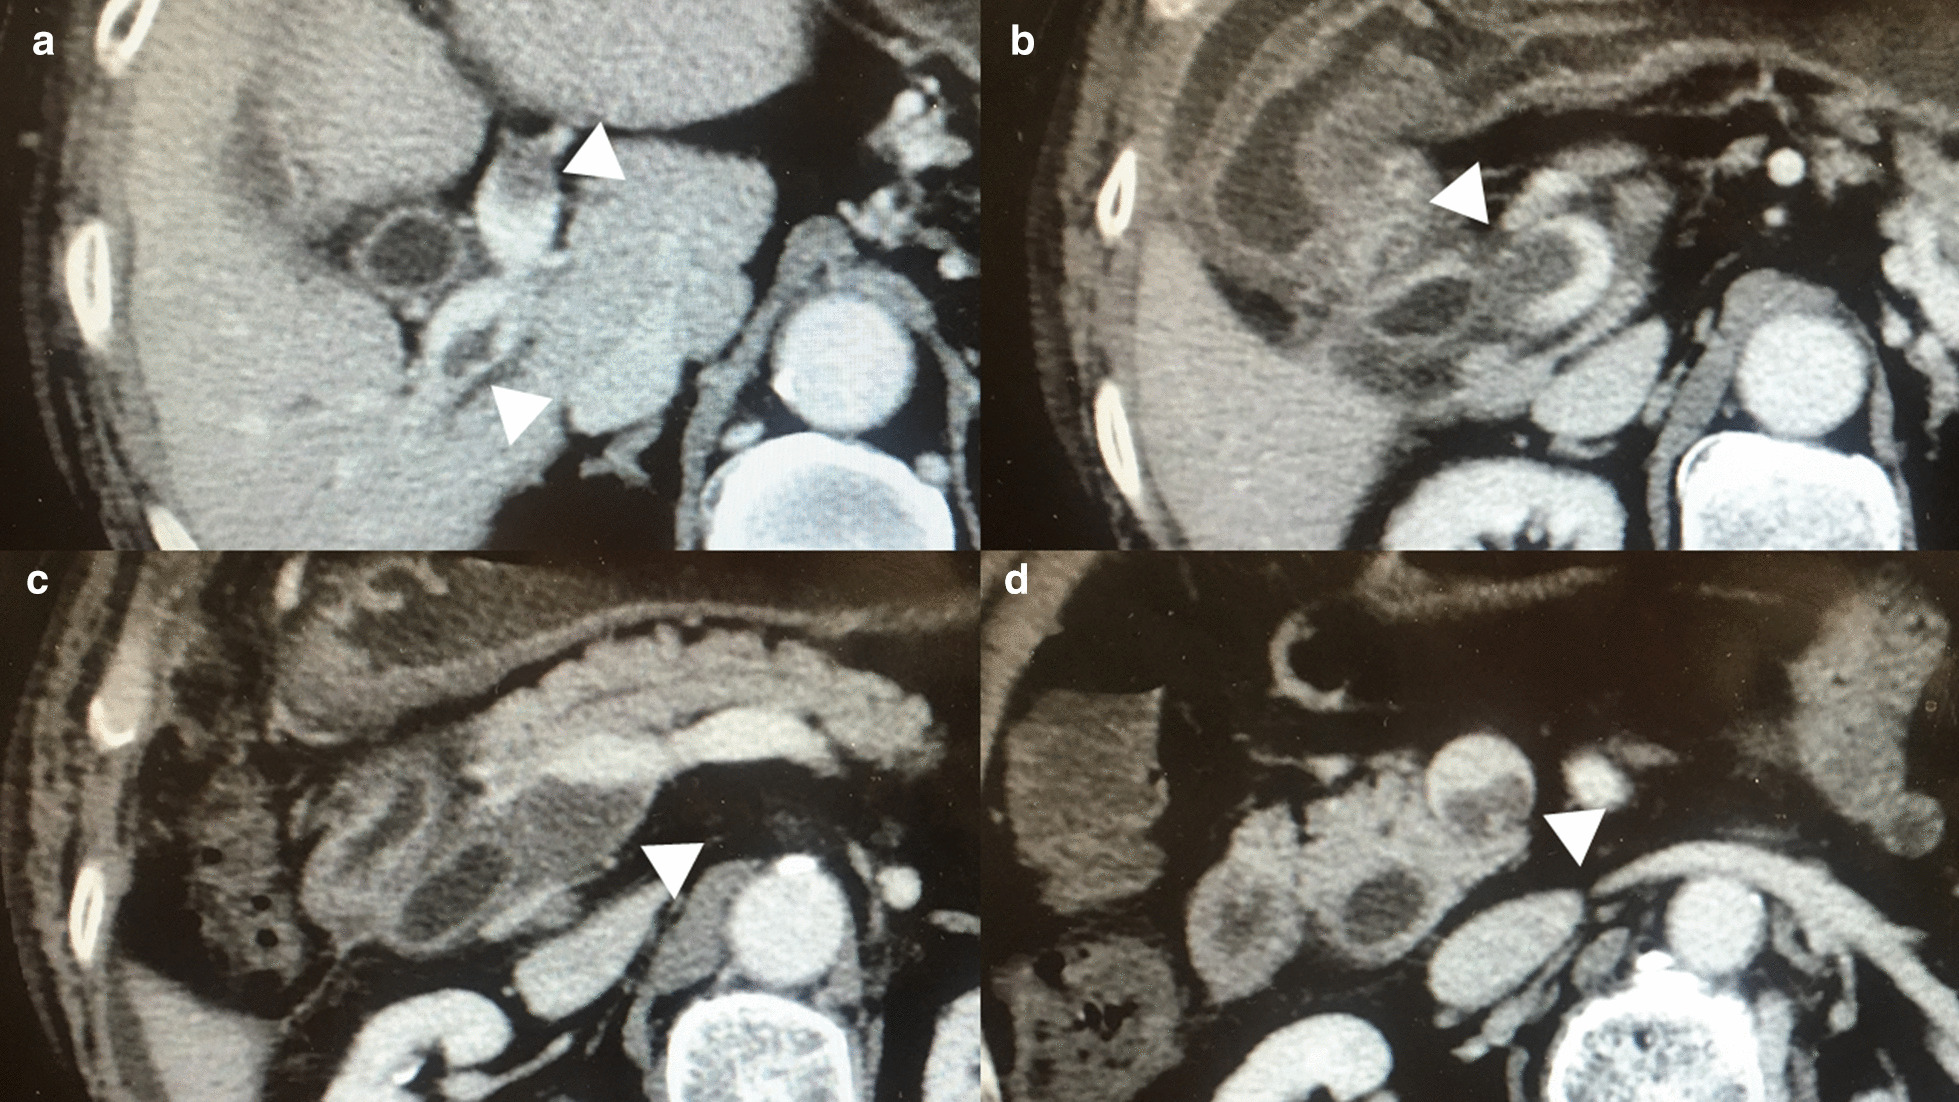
Findings of the portal vein thrombosis on postoperative day 8. Exacerbation of the portal vein thrombosis (PVT) (White arrowheads) was noticed with the findings of contrast-enhanced computed tomography taken on postoperative day 7. The PVT extended cephalically beyond the bifurcation of the right and left portal veins (a). The stenosis caused by the PVT was deteriorated either in the portal trunk (b) or in the confluence of the superior mesenteric vein and the splenic vein (c). Furthermore, the PVT extended much more caudally compared to the preoperative period (d). (*CHD* common hepatic duct; and *CBD* common bile duct)
